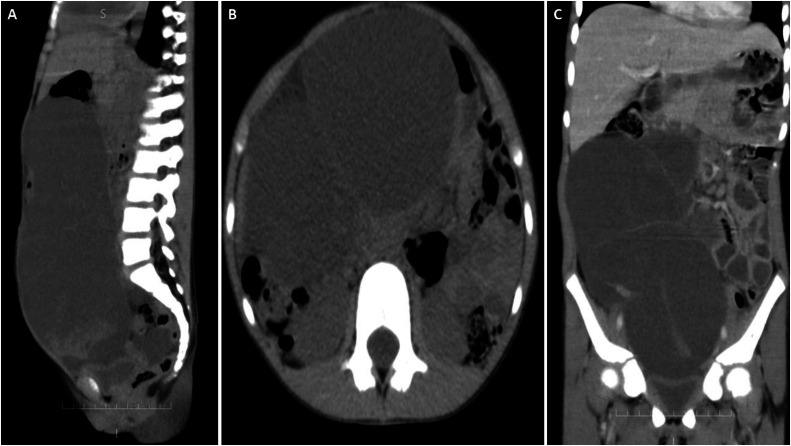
Contrast-enhanced CT of the abdomen and pelvis (portal venous phase) demonstrating a large, multiloculated, cystic lesion with internal septations occupying the abdominal cavity and compressing adjacent organs: (A) Sagittal cross-section. (B) Axial cross-section. (C) Coronal cross-section.
